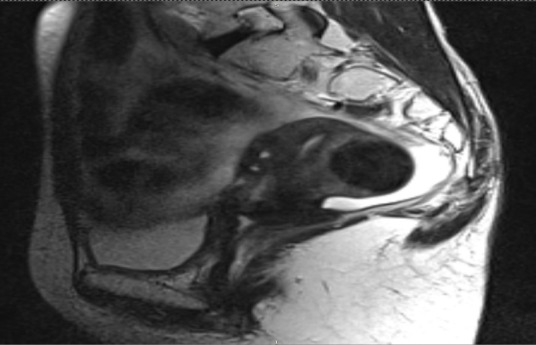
MRI sagittal view of the pelvis of the patient at the end of EBRT (retroverted position of the uterus)
